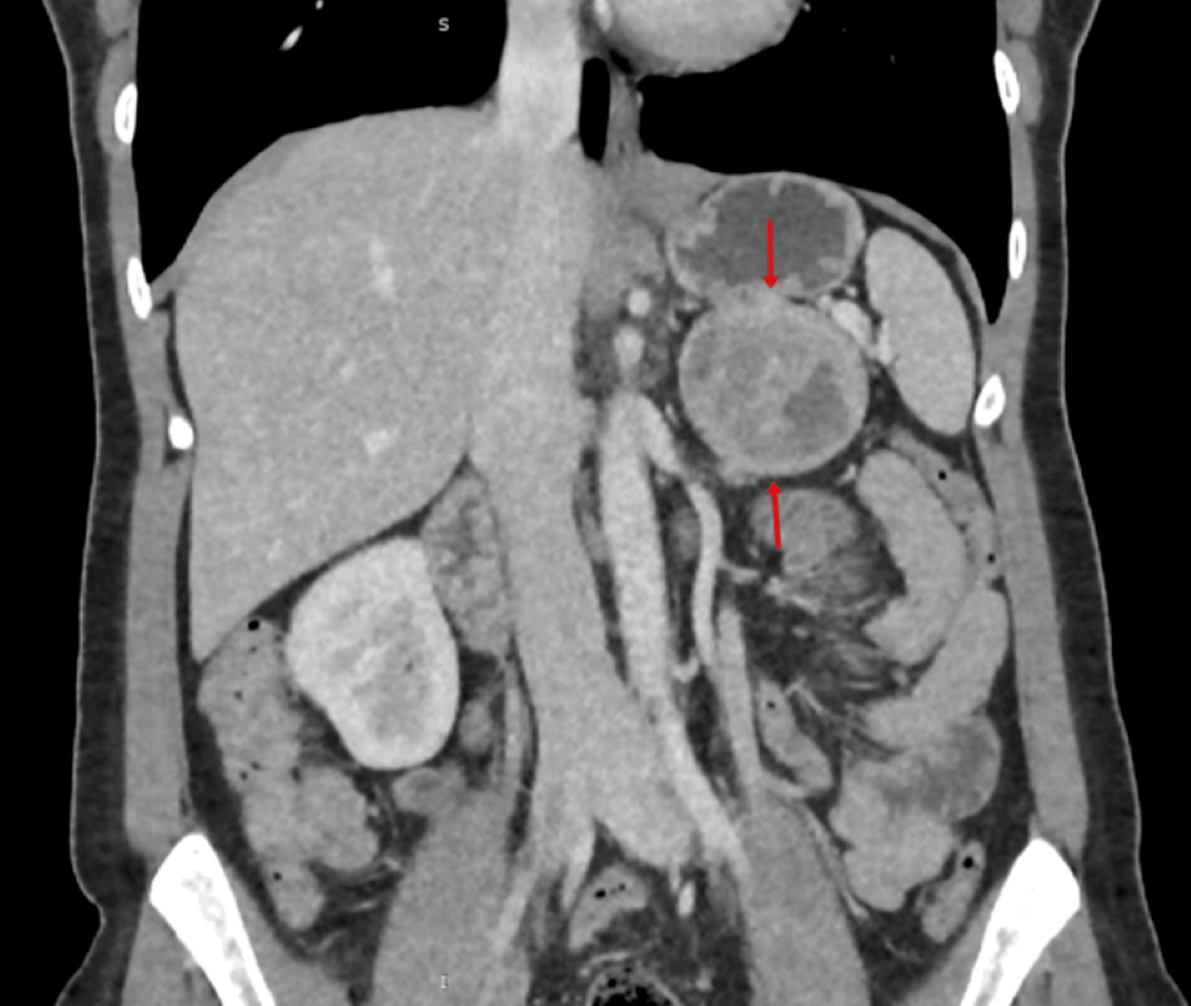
Computed tomography (CT) scan showing a left adrenal mass (arrows)
